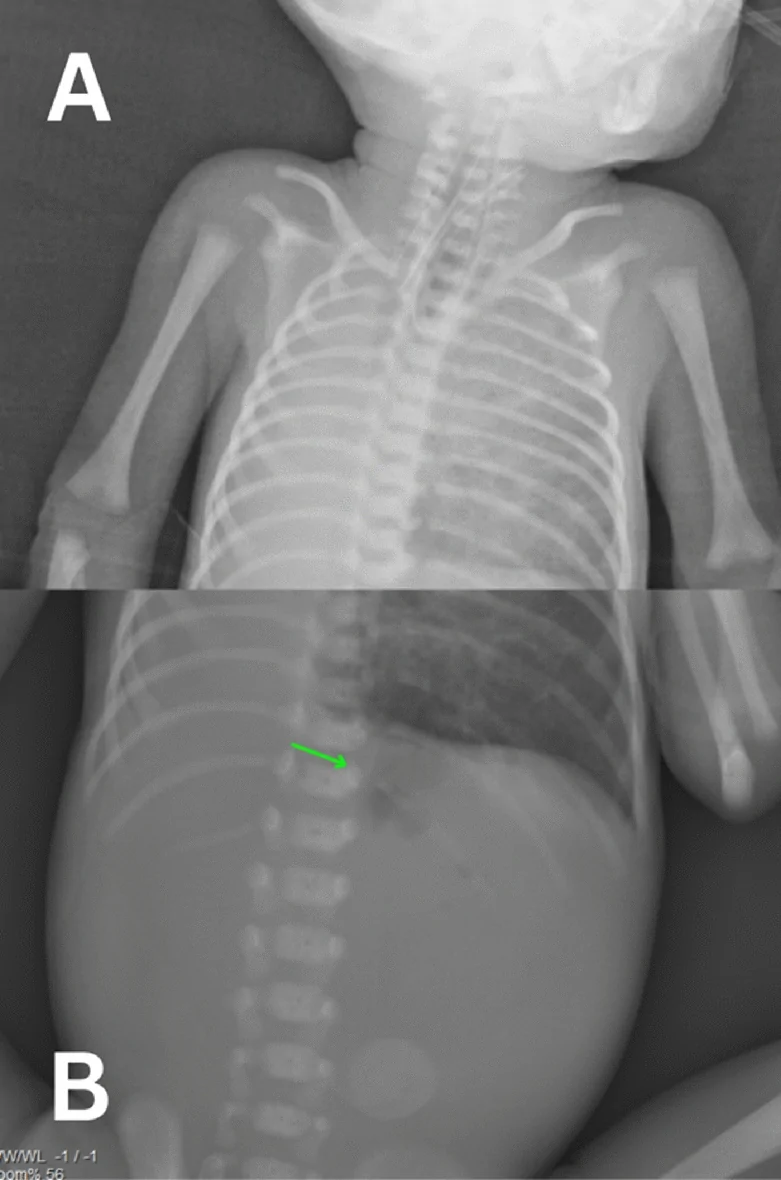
Initial chest radiography at admission (9th day of life). Panel A Plain radiograph demonstrating several critical findings. The endotracheal tube (ETT) is located in situ, whereas the orogastric tube (OGT) is appropriately positioned within the upper mediastinum. There was a complete right-sided white hemithorax with compensatory hyperinflation of the left lung. The cardiac silhouette and mediastinum are shifted to the right. The hyperinflated left lung exhibited diffuse ground-glass and reticulated opacities. Panel B The abdominal portion of the same radiograph demonstrates a normal bowel gas pattern (green arrow)
